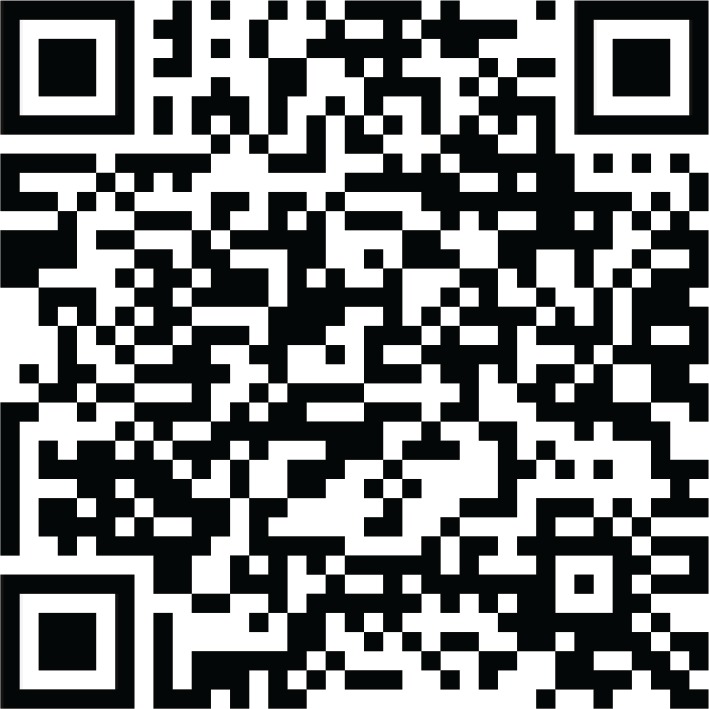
Transthoracic echocardiography: left ventricular pseudoaneurysm neck and anterior wall hypokinesis.
